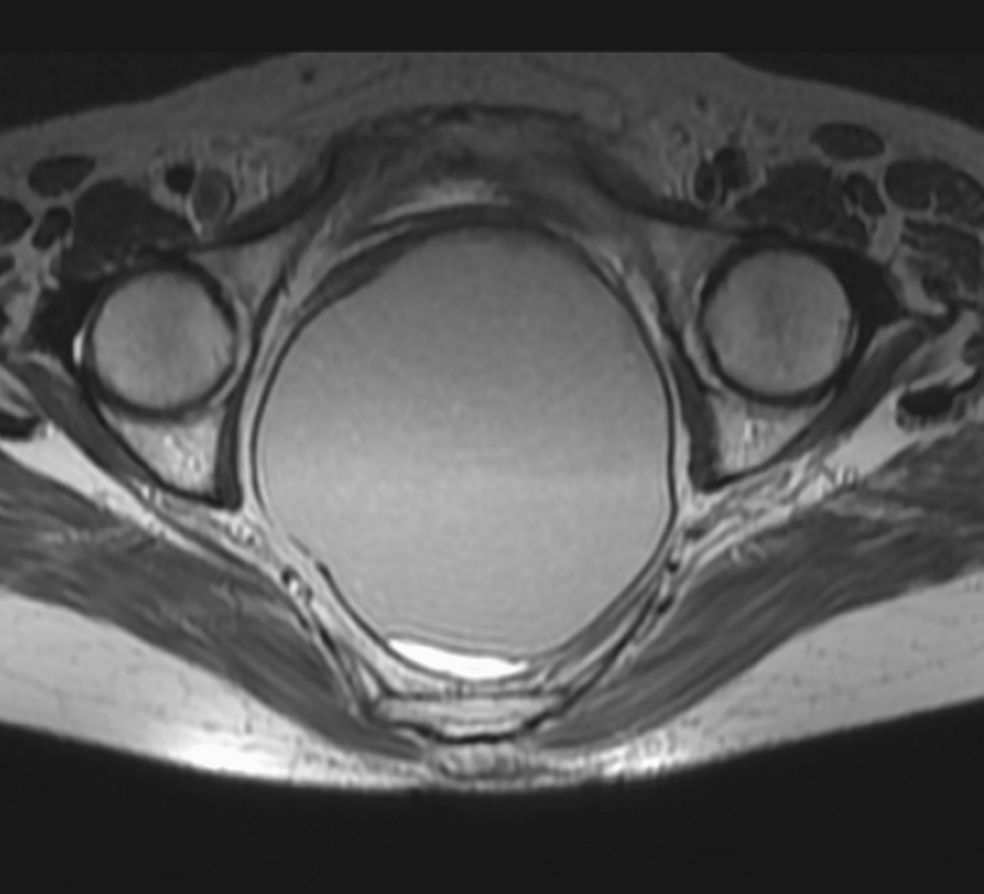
MRI of the swelling revealing a cystic lesion
